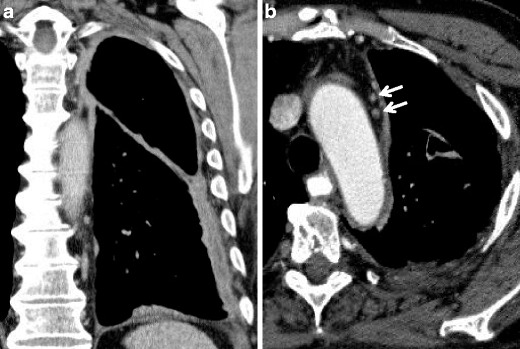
Stage I of MM; contrast-enhanced CT scan: coronal (a) and axial (b) view. Diffuse circumferential thickening of the thoracic, mediastinal and diaphragmatic pleura involving even the fissure. No extra-pleural infiltration or significant lymphadenopaties are observed (only two small lymph nodes are reported—*white arrows*)
